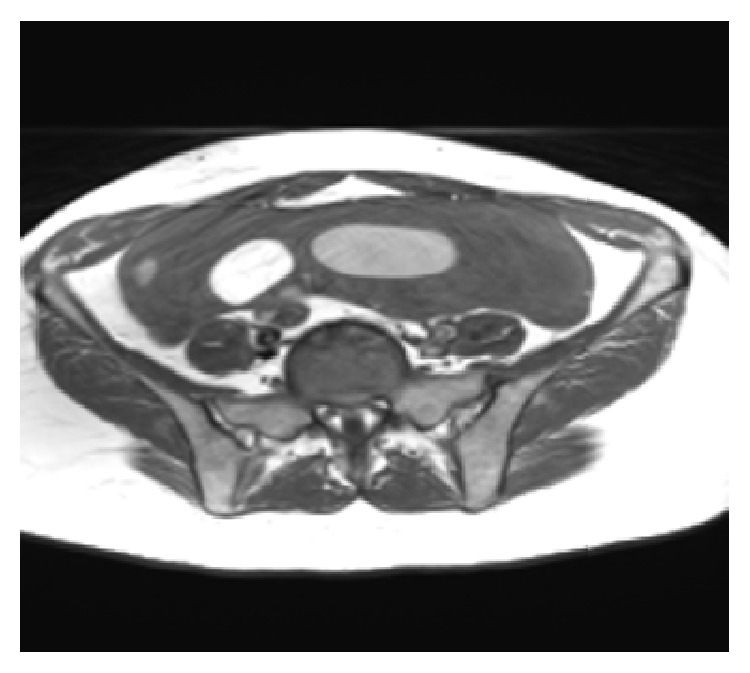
MRI picture of the patient.
